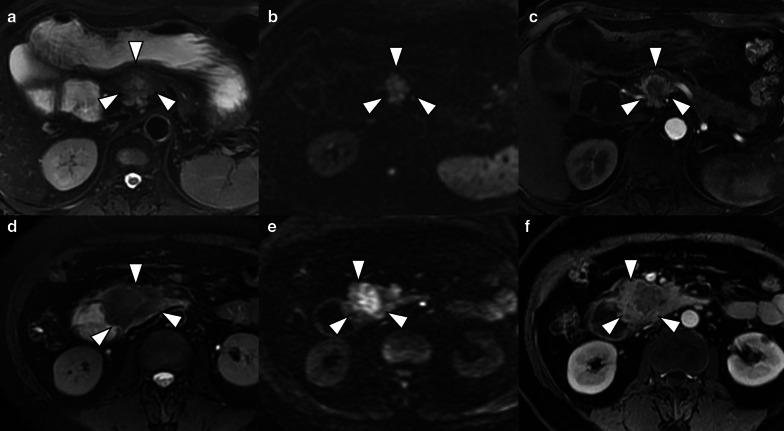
NF-PNETs reveal irregular characteristics on T2WI (left), DWI (middle) and arterial phase (right) images. The tumor shows ill-defined nodular tumor–pancreas interface with infiltrative to adjacent normal pancreatic parenchyma on (a–c). Highly infiltrative tumor with confluent multinodular growth and lacking demarcation is detected on (d–f), showing directly penetration to the duodenum with pathologic proven
